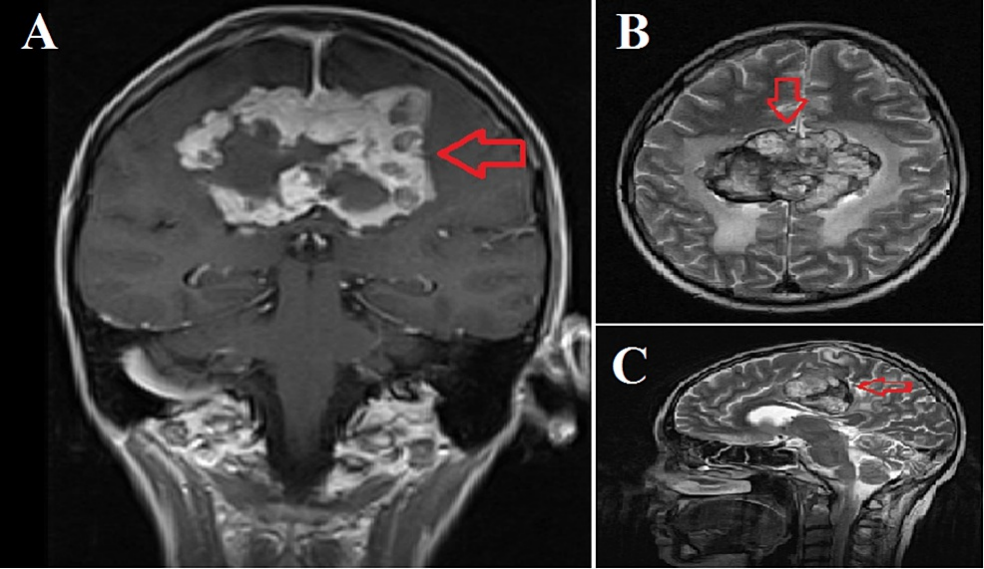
Magnetic resonance images of the cranial metastatic mass A: Coronal section view of the cranial metastatic mass. B: Transverse section view of the cranial metastatic mass. C: Sagittal section view of the cranial metastatic mass. Red arrow: the cranial metastatic mass.
